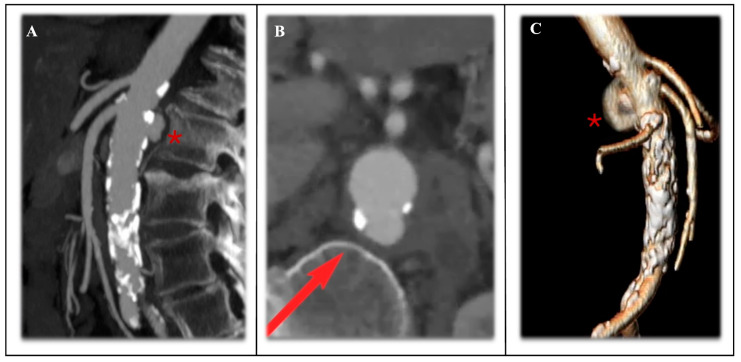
Preoperative computed tomography angiography (CTA) of the para-visceral penetrating aortic ulcer (PAU) positioned at the level of the superior mesenteric artery (SMA) in MPR projection ((A) red asteriks), in planar 2D vision ((B) red arrow, in the posterior portion of the aorta) and in the 3D volume rendering recontruction ((C), red asterisk).
